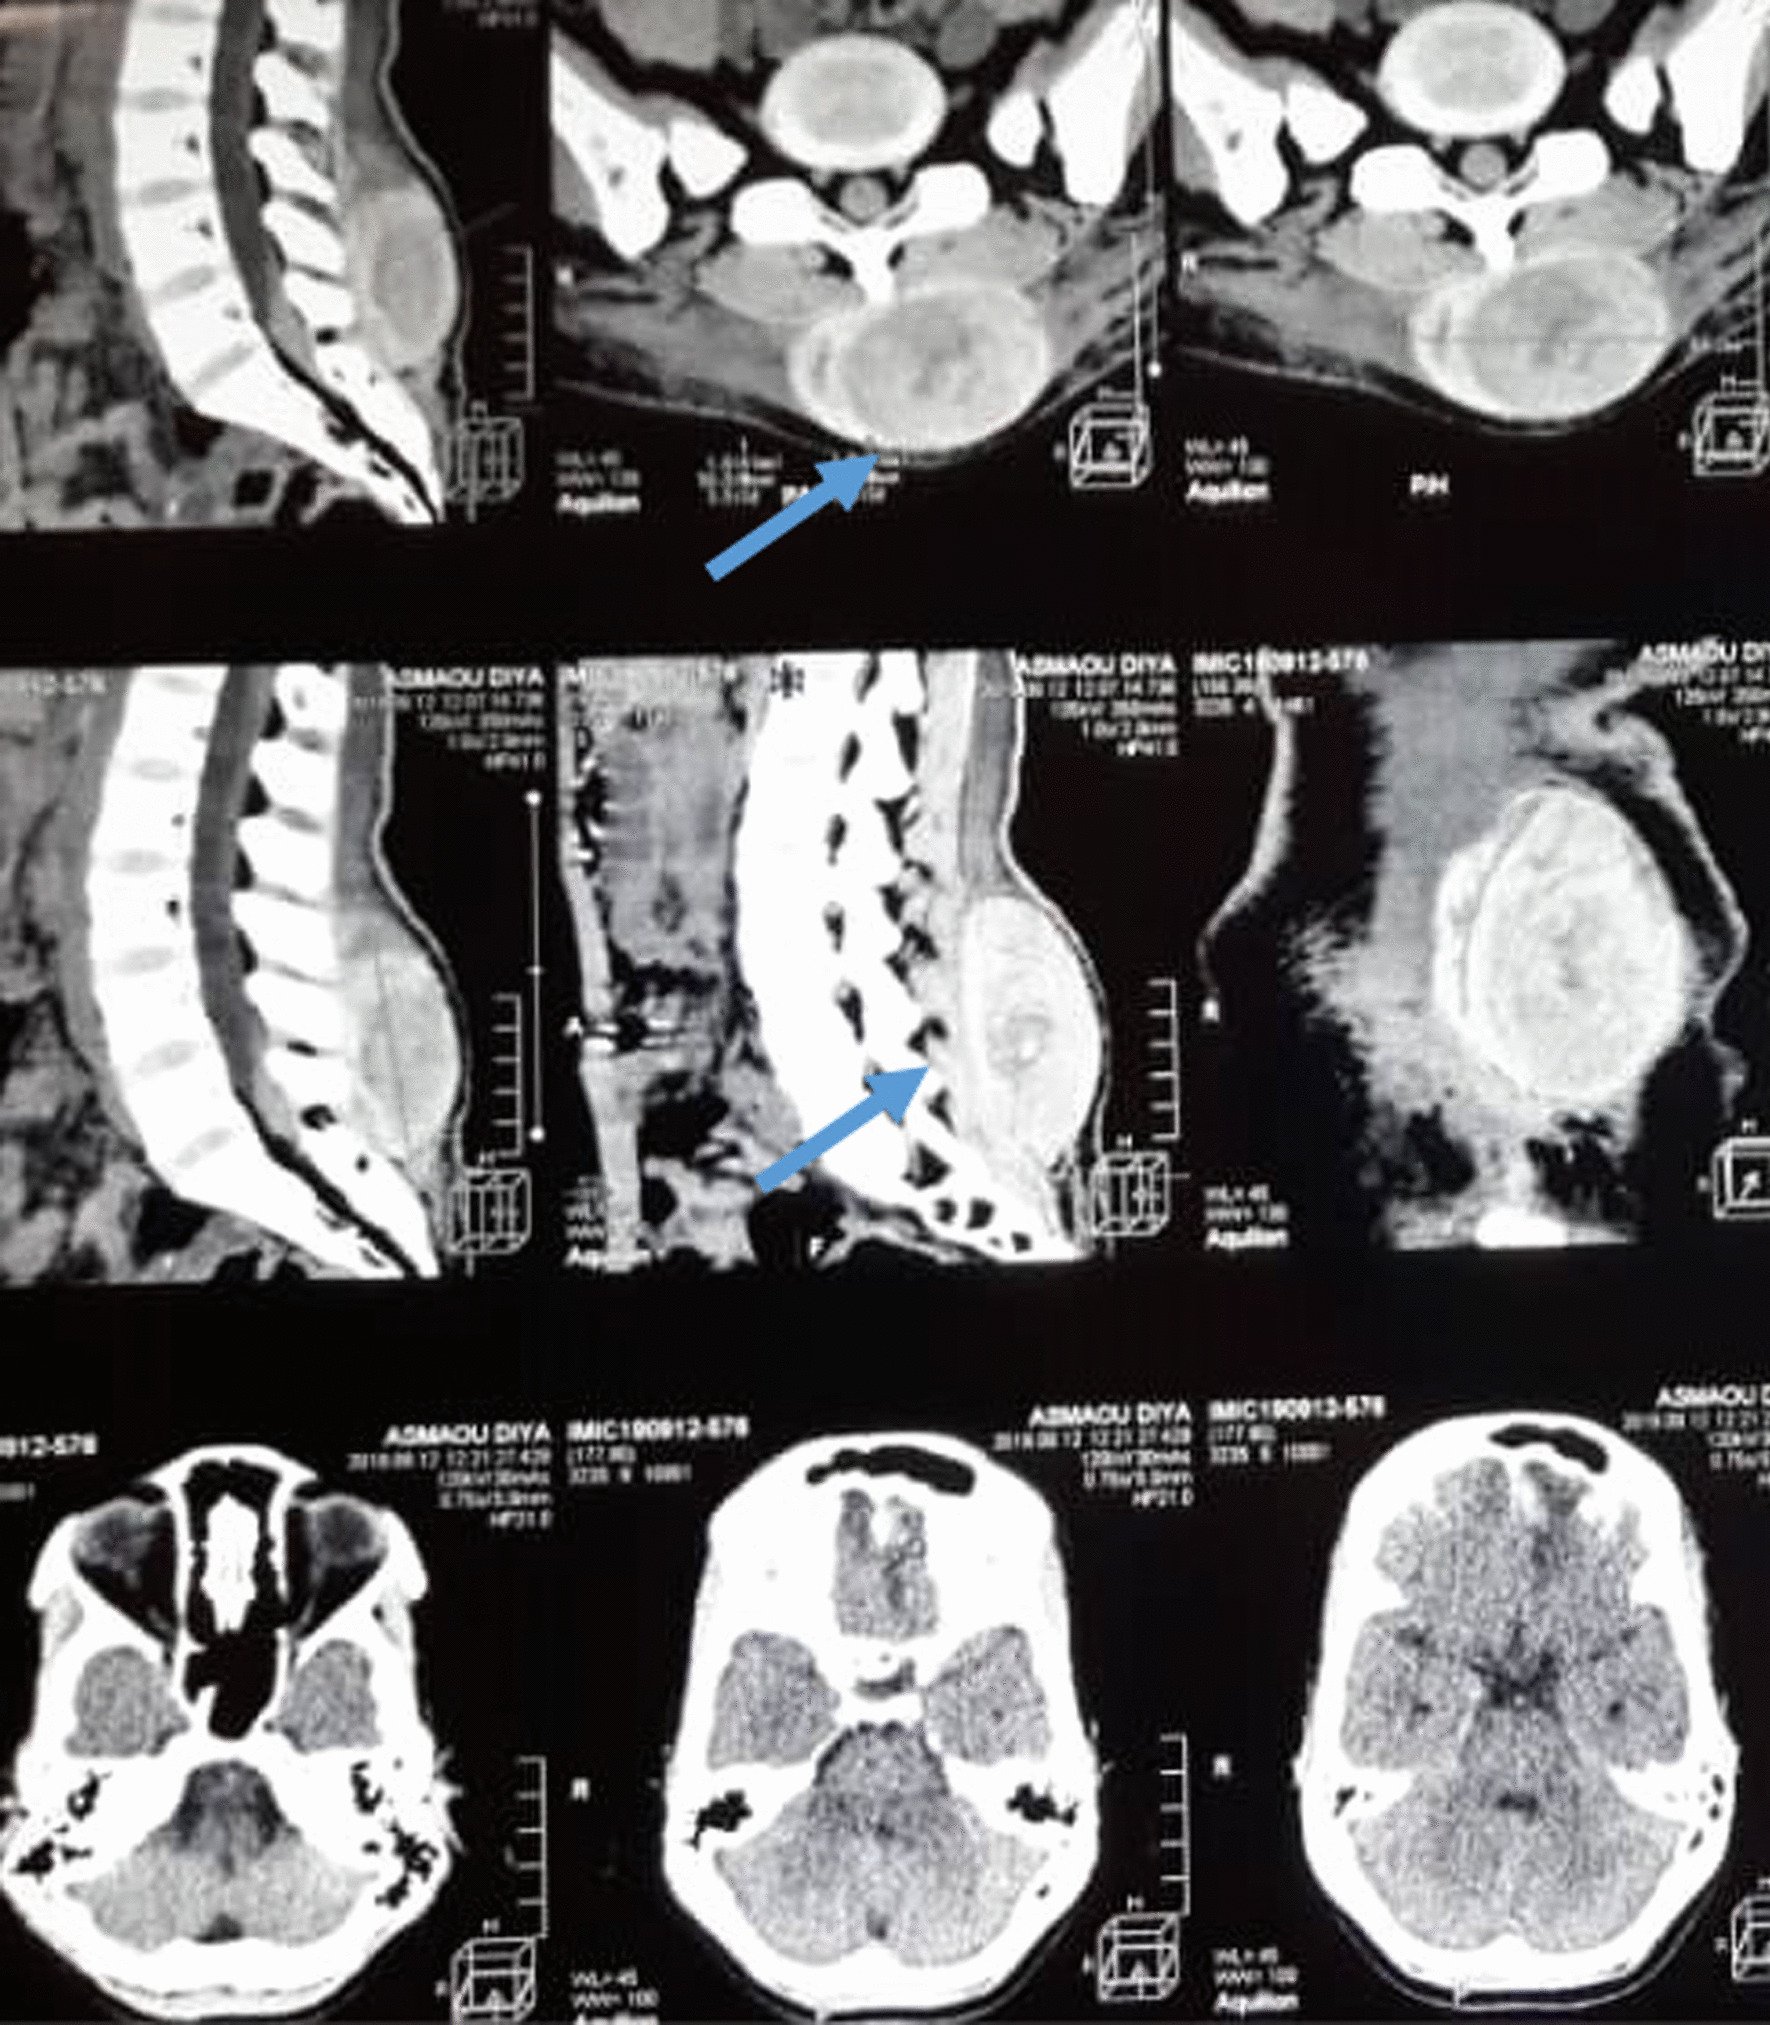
Cerebral CT: left frontal concussion lumbar. CT: left paraspinal hyperdense mass. The arrows are pointing the Morel-Lavallée Lesion
